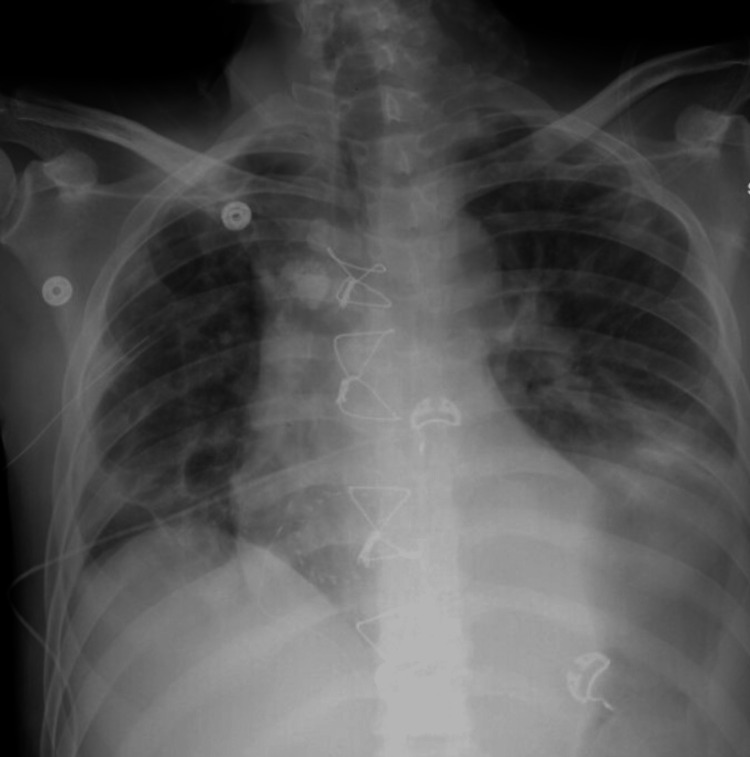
Chest X-ray showing improved pneumothoraces and aeration of both lungs.
